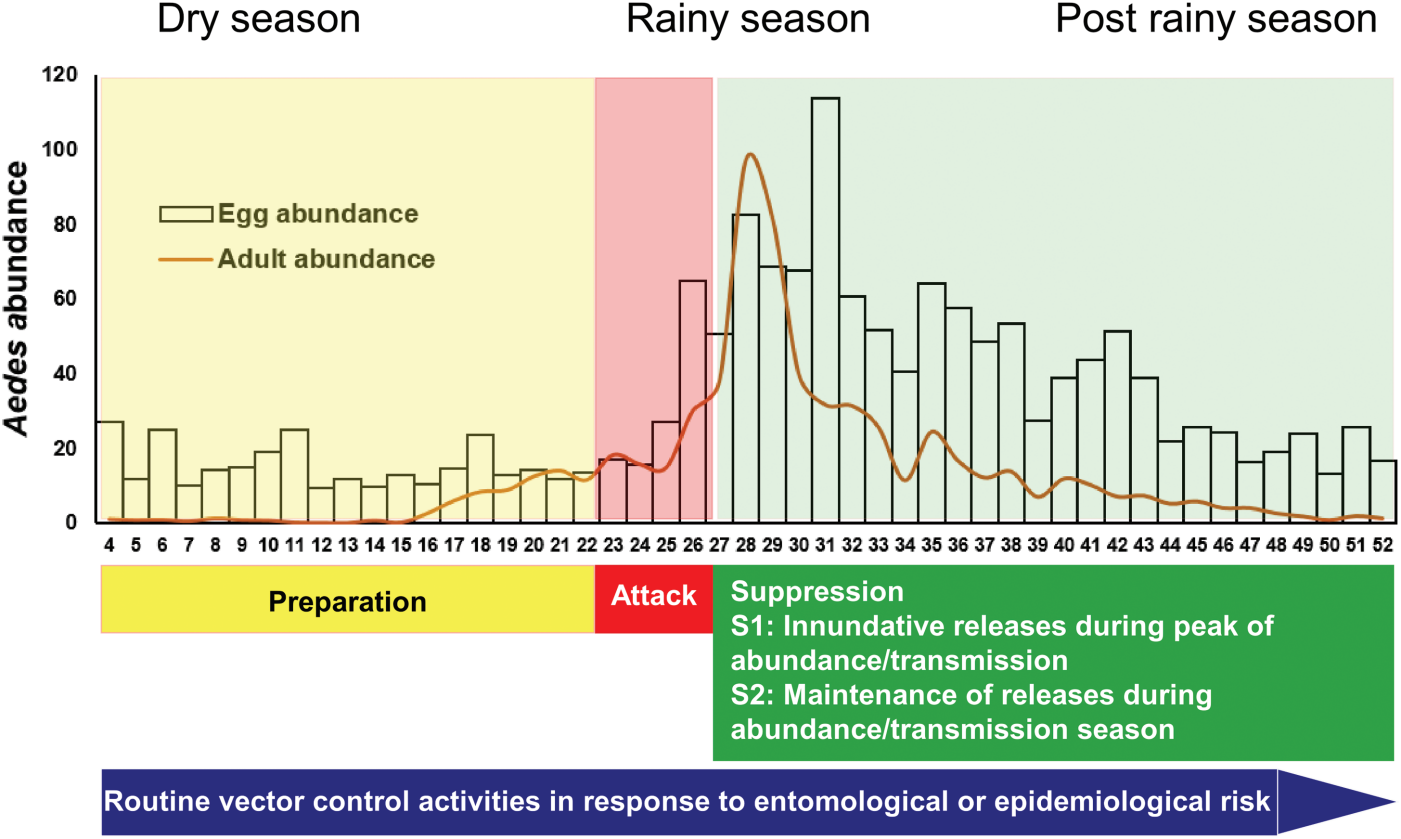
Proposed pilot IVM plan combining ‘traditional *Aedes* control’ and IIT/SIT based on the release of X-ray irradiated male *Ae. aegypti* carrying *Wolbachia* for population suppression. The strategy will be structured in three phases: Preparation: with community sensitization and engagement, and baseline entomological studies; Attack: initial traditional vector control; and Suppression phase: S1: inundative releases during peak of abundance/transmission and S2: maintenance of releases during abundance/transmission. Curves of egg and adult abundances are based on the average results from both study sites.
